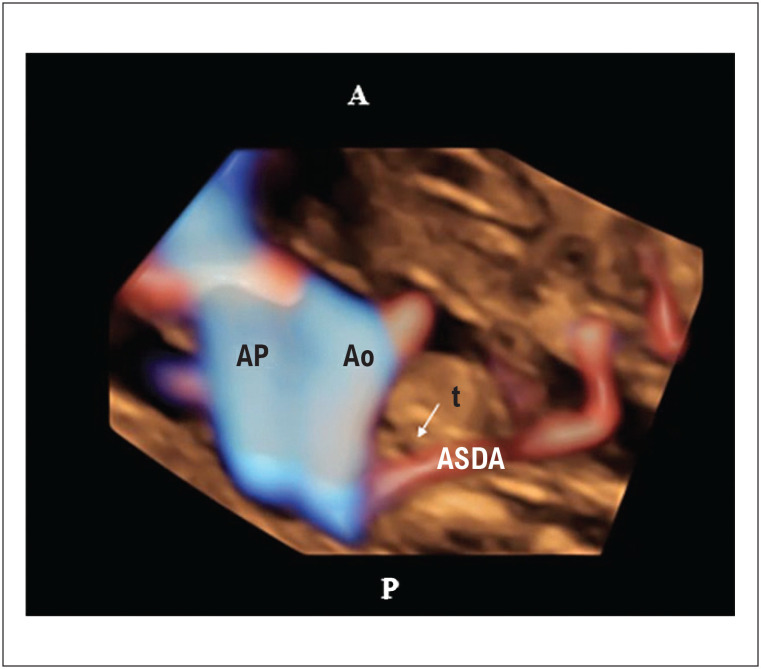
Ultrassonografia tridimensional com HDlive flow e modos Silhouette da visualização de três vasos e traqueia (3VT) mostrando uma artéria subclávia direita aberrante (ASDA) de um feto com 20 semanas e 6 dias. A ASDA surge da aorta como o quarto vaso. Foi descrita como um marcador de risco para anomalias cromossômicas, como a trissomia do 21. Observa-se que a ASDA passa posteriormente à traqueia na visualização 3VT. A artéria subclávia direita normal é um vaso em forma de S que passa anteriormente à traqueia. Ao: aorta; AP: artéria pulmonar; ASDA: artéria subclávia direita aberrante, t: traqueia; P: posterior; A: anterior.
